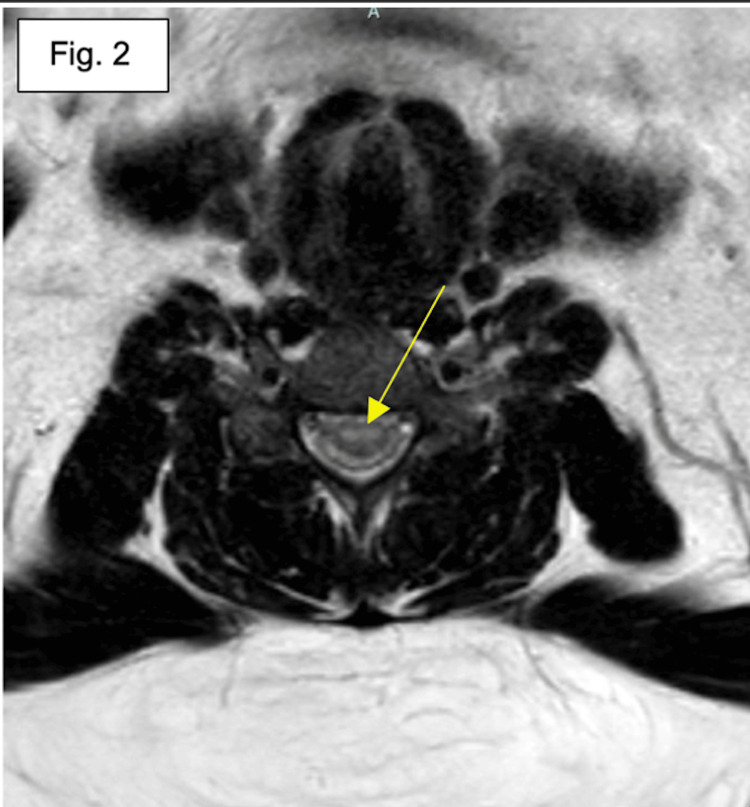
Axial T2-weighted cervical spine MRI at the C5 level demonstrating anterior column hyperintensity (arrow).
